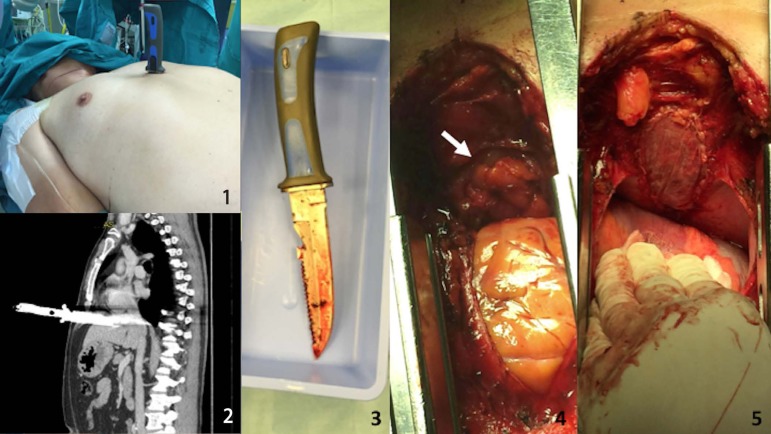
1 - Knife penetrating the chest; 2- preoperative CT-scan; 3 - knife
